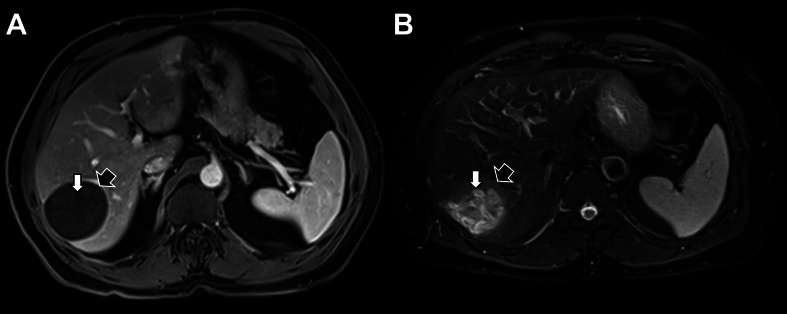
Magnetic Resonance Imaging (MRI) of the *Echinococcus ortleppi* hydatid cyst. Typical 4CE cyst imaging showing a floating membrane (white arrows) in a solid matrix (black arrows), in T1 (A) and T2 (B) acquisition.
